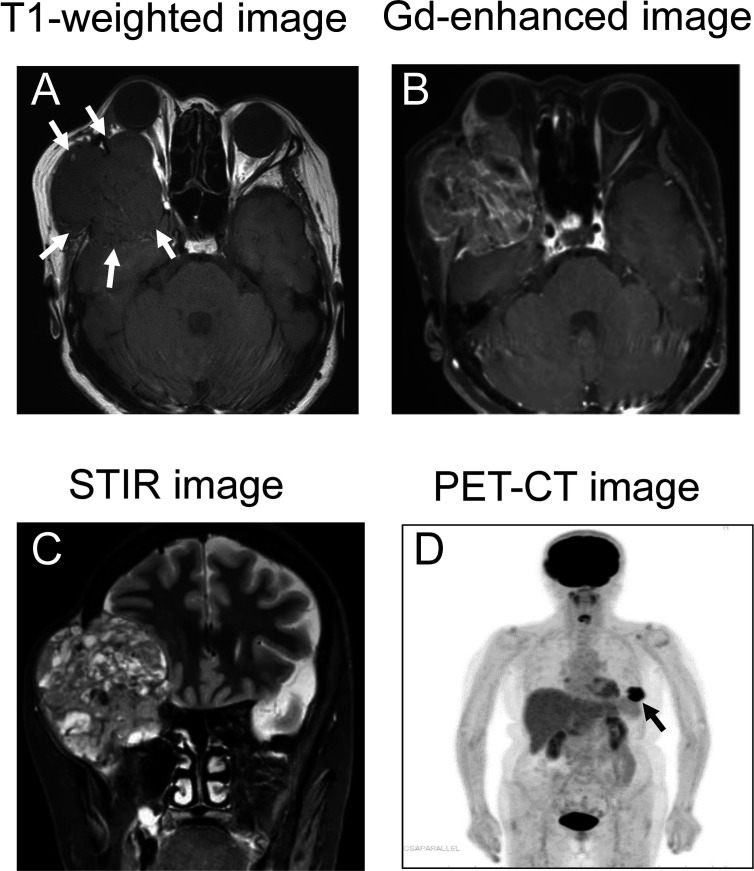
MRI and PET-CT image. (A) The tumor displaces the extraocular muscles and optic nerve of the right eye, and brain parenchyma as indicated by arrows (T1-weighted image). Arrows indicate the tumor. (B) The tumor showed contrast-enhanced with gadolinium. (C) STIR imaging shows a non-homogeneous giant tumor compressing the brain. (D) PET-CT demonstrates integration of FDG coincident with the left breast, as shown by the arrow.
